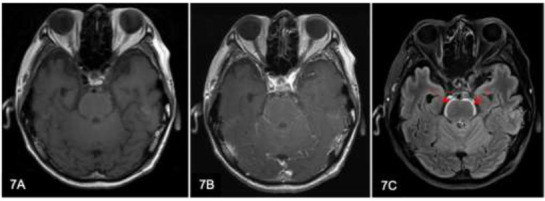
A 52-year-old man with lung adenocarcinoma underwent an MRI brain for staging purposes. Axial T1-weighted (w) (A) and T1w post-contrast (B) MRI brain images show no abnormal enhancement. Axial FLAIR (C) image demonstrates hyperintensity along the surface of the brainstem (arrows). This appearance is referred to as the ‘bloomy-rind sign,’ which is attributed to the spread of cancer cells to the surface of the brain in patients with lung adenocarcinoma undergoing treatment with tyrosine kinase inhibitors.
